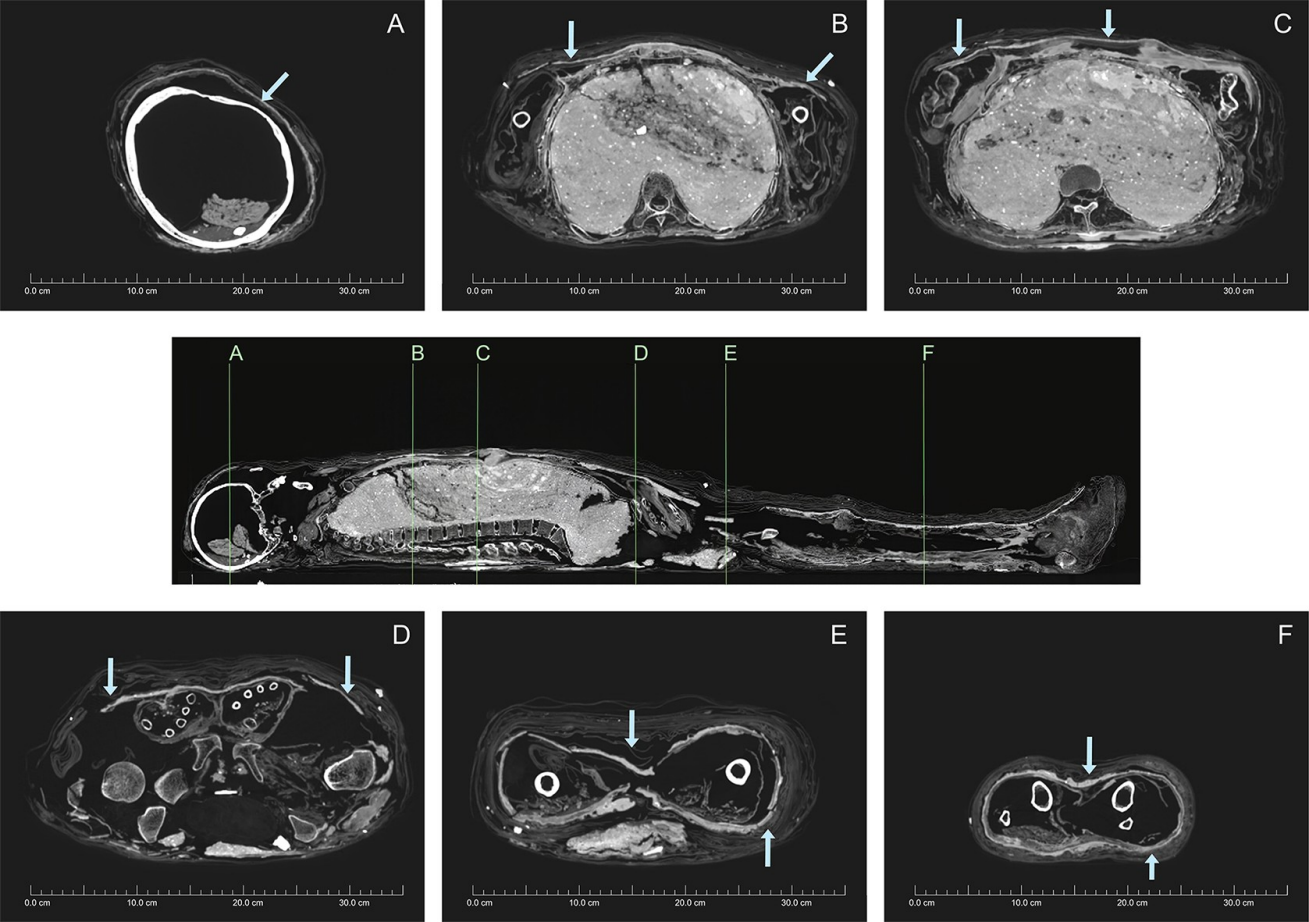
Axial CT images of mummified individual (NMR.27.3) showing transverse views of the carapace at different locations. The carapace is a thin white line under the wrappings (arrowed) (courtesy Chau Chak Wing Museum and Macquarie Medical Imaging).
